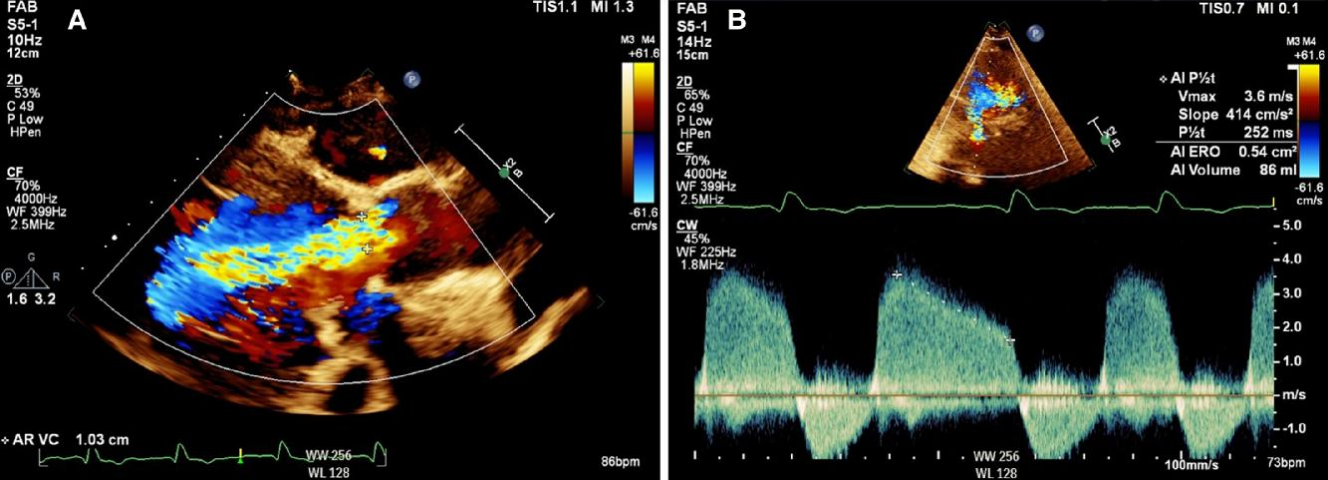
Transthoracic echocardiography demonstrating severe aortic regurgitation. (*A*) Parasternal long axis (vena contracta 1 cm); (*B*) continuous wave (CW) Doppler through the aortic valve in the apical 5-chamber view.
